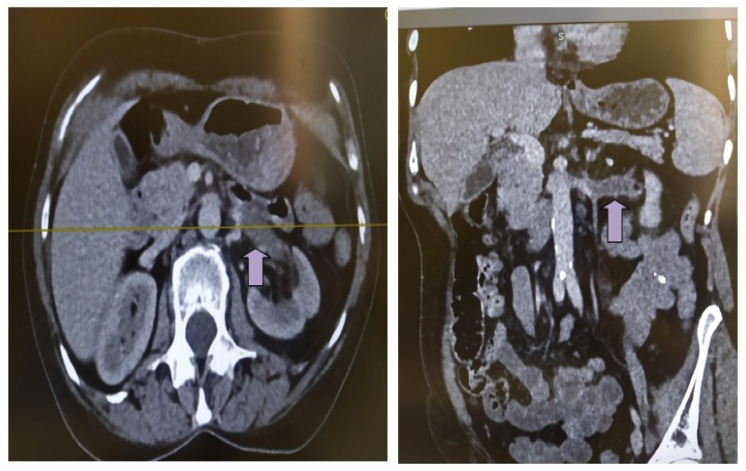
Abdominal CT scan/angiography during follow-up (1 month after renal vein thrombosis diagnosis) revealed renal vein re-tunneling (arrow) (axial and frontal level).
